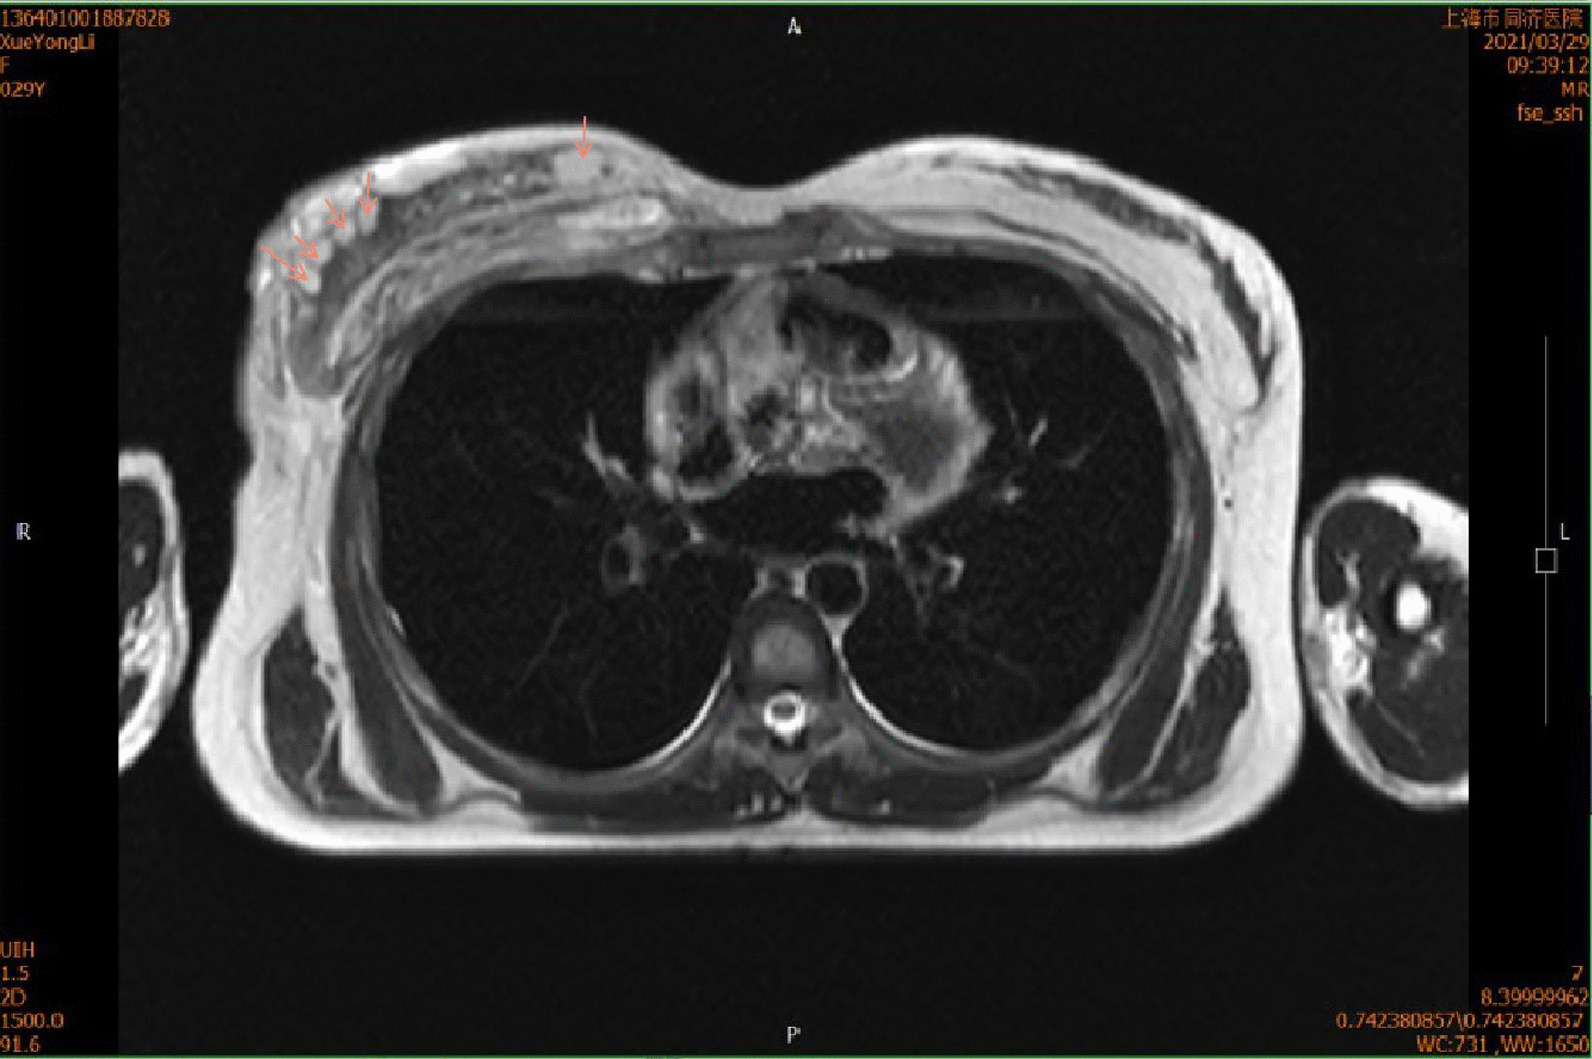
Magnetic Resonance Imaging (MRI) demonstrated: High signal intensity on T2-weighted images involving soft tissues in the region of the right pectorals major muscle, right shoulder and right upper arm was detected; multiple nodular-like high signal intensity (→) were shown in right mammary gland, which became hypointensity after lipid suppression
